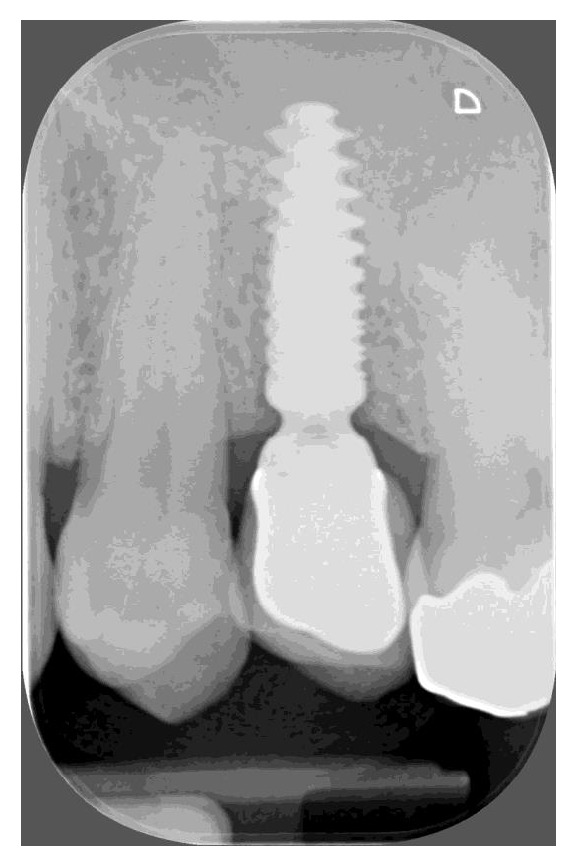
Radiographic 5-year follow-up of a single restoration. Some bone growth over the shoulder of the implant can be detected.
